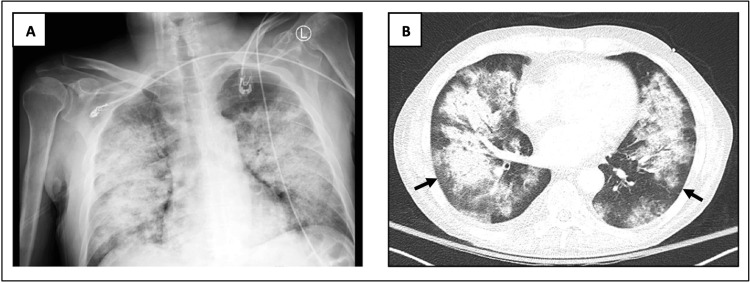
X-ray (A) and CT scan (B) of the chest on admission The images showed bilateral pulmonary infiltrates sparing the periphery (arrows) CT: computed tomography
